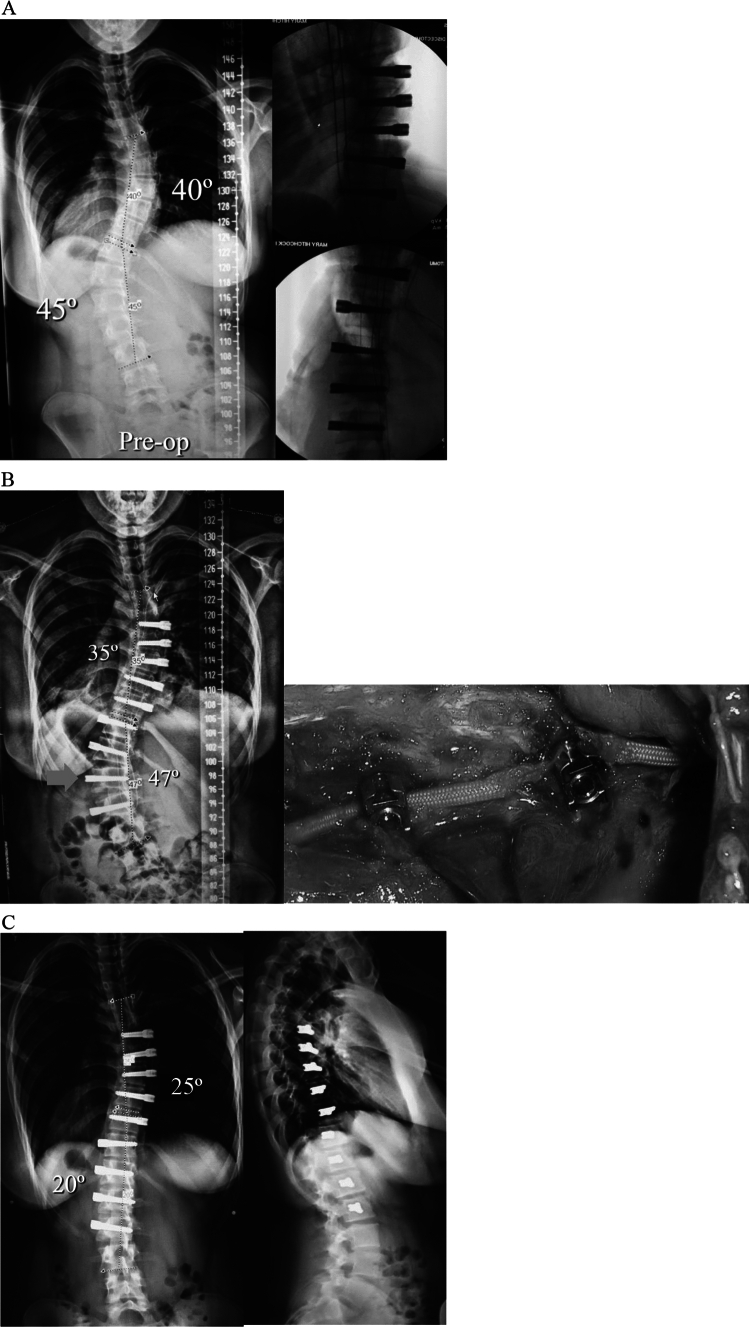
A Preoperative posteroanterior radiograph of a 14 + 11-year-old female with a Lenke 6C curve pattern involving a 40º curve right T6-T11 and 45º curve left T11-L3 at Risser 4 and Sanders 6 with significant correction of both curves to < 20º demonstrated on intra-operative fluoroscopy. B Postoperative posteroanterior radiograph after an audible pop was heard while ambulating on POD #2 demonstrating loss of correction of both curves but most significantly spanning T12-L2. An intra-operative photograph taken during revision surgery demonstrated tether rupture at the L1 screw. Analysis of the explanted Dynesys PET cord confirmed focal damage at the L1 screw site most likely related to overtightening of the Dynesys set screw with a non-torque-limiting screwdriver. C Postoperative posteroanterior and lateral radiographs demonstrating improvement of both curves to 25º and 20º, respectively, after immediate revision of the ruptured thoracolumbar tether only. Curve corrections were maintained at 2 year follow-up without further tether rupture issues
